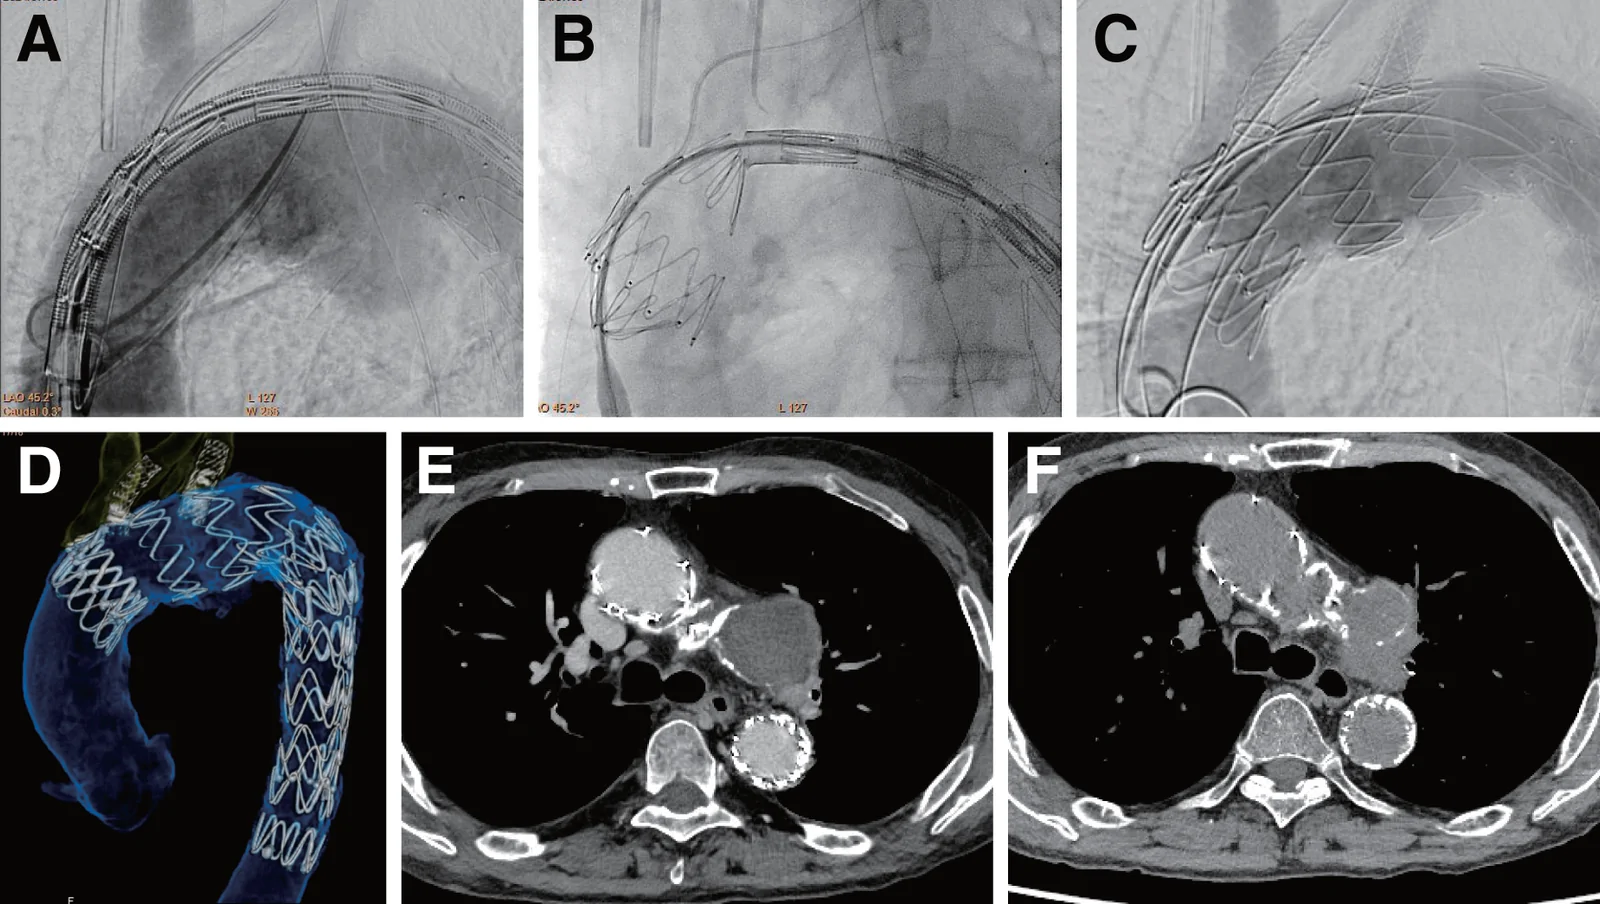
Intraoperative findings. (A) DSA before deployment of the physician-modified endovascular Zenith TX Alpha stent graft. (B) The device is deployed into the fenestration of the left common carotid artery, and a 4-Fr catheter is tried to cannulate into the fenestration. (C) Final DSA after VBX stent-graft deployment. (D) Postoperative contrast-enhanced CT shows no endoleaks. (E) Postoperative contrast-enhanced CT (axial). (F) CT at 18 months postoperatively demonstrating favorable sac regression. DSA, digital subtraction angiography; CT, computed tomography
